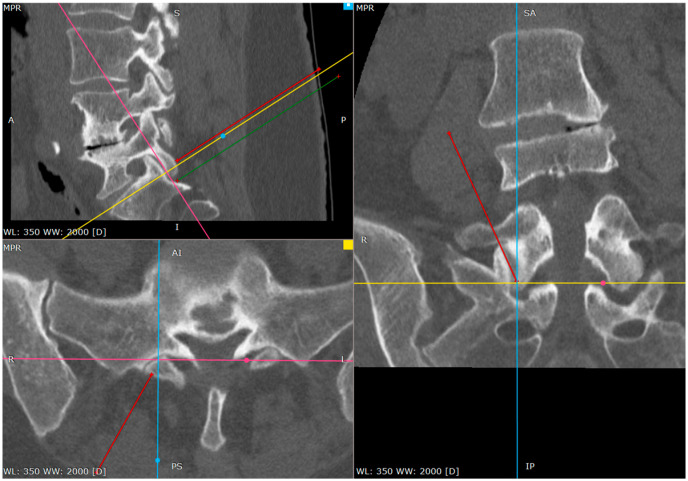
The primary target point—the inferior part of the facet (a CT tri-plane reconstruction).
